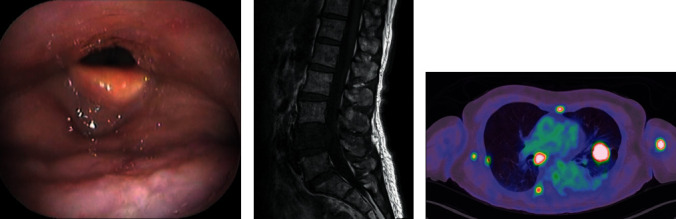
At 20 months after treatment, (a) an endoscopic examination of the pharynx showed that there was no recurrence at the base of the tongue. (b) Lumbar MRI T1W1 showed a metastatic lesion in the L4 vertebra. (c) PET-CT showed multiple metastases in the bilateral lungs, right hilum, right axillary lymph nodes, and some bones.
